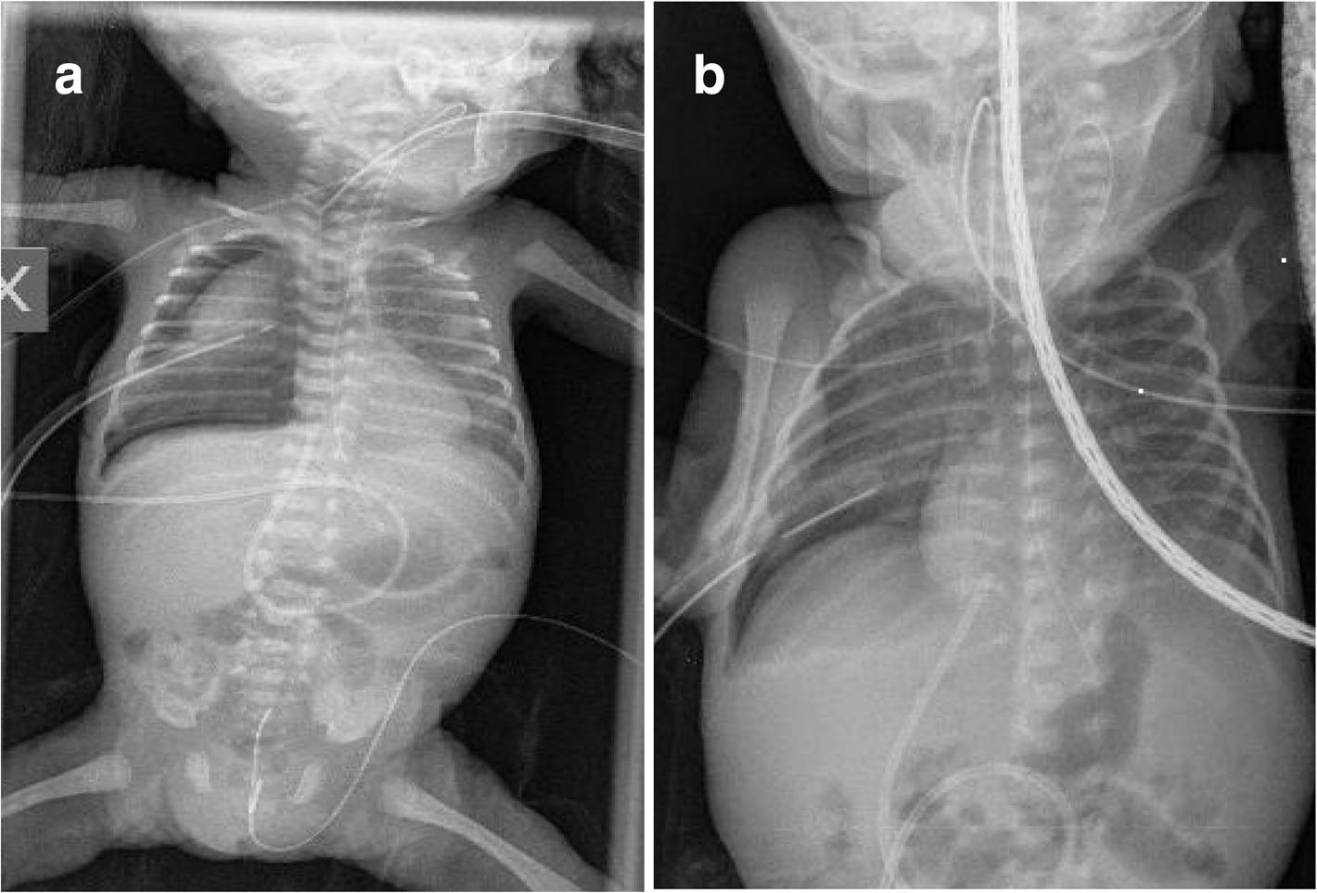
PTX in the first and fourteenth day of life. Chest x-ray showing right-sided PTX in the first day of life (a) persisting in the fourteenth day of life (b)
